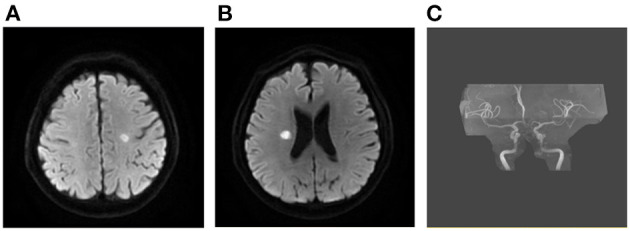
DWI of patients with cerebral small vessel disease. A 51-year-old man had developed left-side central facial palsy, weakness of left limbs, and right hand numbness. MRI showing bilateral supra-tentorial ischemic lesions (A, B). MRA did not show obvious large artery stenosis (C), and no other etiology was found. DWI, diffusion-weighted imaging; MRI, magnetic resonance imaging; MRA, magnetic resonance angiograph.
